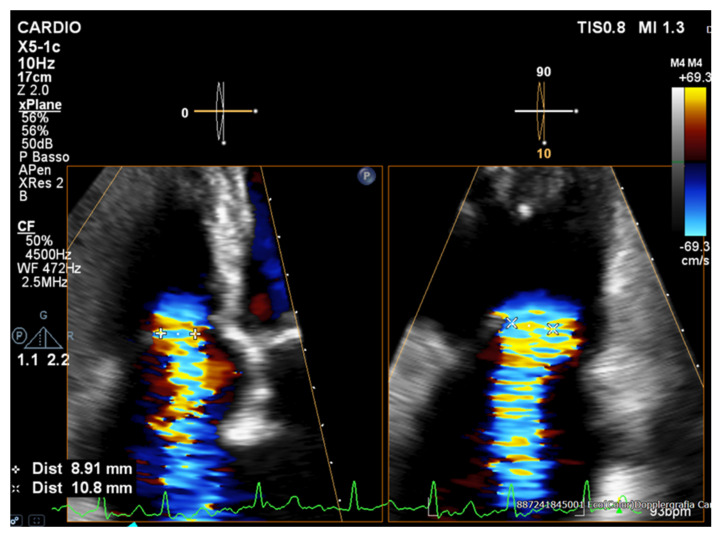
Biplane measurement of the vena contracta for tricuspid regurgitation quantification. The image shows a right ventricular (RV) focus view on transthoracic echocardiography (TTE) with biplane measurement of the vena contracta (VC) for tricuspid regurgitation (TR) quantification.
